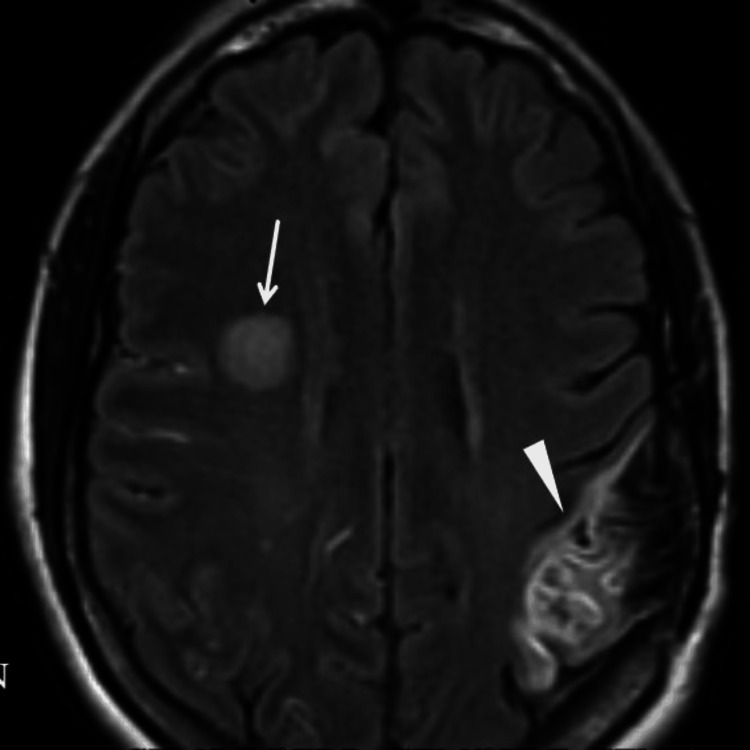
Brain MRI shows a significant lesion in the right centrum semiovale, likely related to an embolic acute ischemic infarct (arrow) and old wedge-shaped hyperintensities in the left parieto-occipital region, suggestive of an old area of ischemic infarct (arrowhead).
